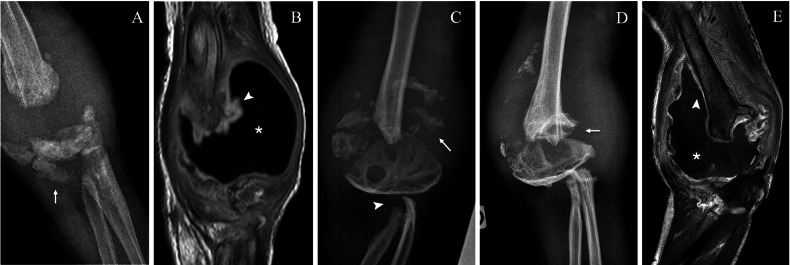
Neuropathic arthropathy of the right elbow of the same boy as in Fig. 4 with bilateral involvement of the elbows. Anteroposterior (AP) radiograph (A) and coronal T1-weighted post-gadolinium MRI (B) at 2 years of age during an episode of septic arthritis complicating the pre-existing NA, showing fragmentation of the distal humeral epiphysis (arrow), synovial thickening and enhancement (arrowhead) and joint effusion (asterisk). AP radiograph at age 6 years (C) shows partial healing of the humeral epiphysis with persistent fragmentation (arrow) and resorption of the proximal radius and ulna (arrowhead). AP radiograph (D) and coronal T1-weighted post-gadolinium MRI (E) show distal humeral pseudoarthrosis (arrow), joint affusion (asterisk) and synovial thickening and enhancement (arrowhead) with a sterile joint fluid aspiration
